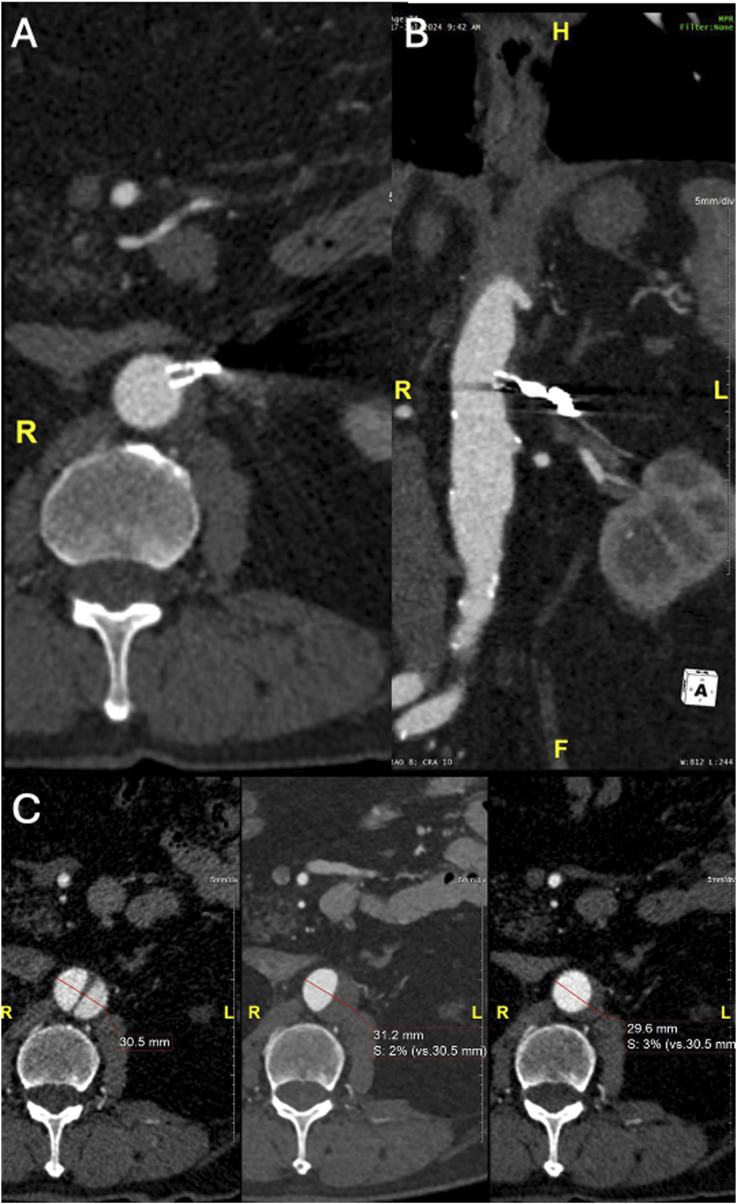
Six-month Follow-up CTA showing complete FL thrombosis and comparative aortic diameter of pre–post procedure and FU CTA. (A) Axial CTA (B) MPR CTA in an obliqual coronal plane (C) Comparative aortic diameter from right to left of pre–post procedure and FU CTA at the level of the distal tear.
